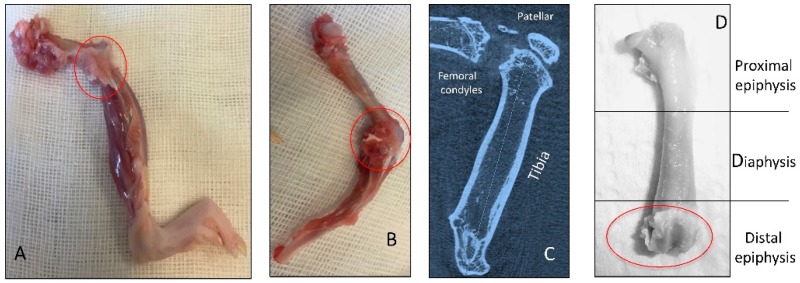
Representation of *in vivo* explanted knee join bones (red circles). (A,B) Knee OA bones joint after explantation, not cleaned of soft tissues; (C) X-ray microtomography imaging of the OA knee bones joint; (D) OA femur cleaned of soft tissues.
